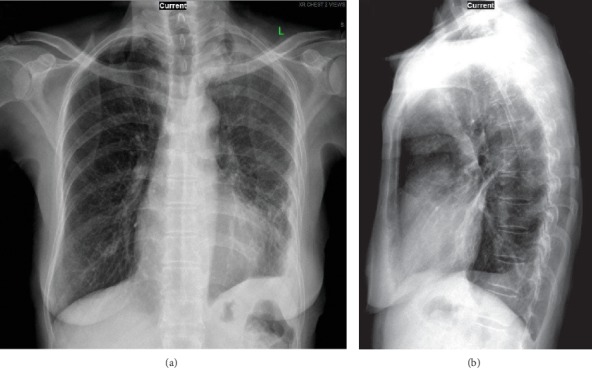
Chest X-ray images of the improvement of the opacity at the left lower hemithorax at week 3 of intravenous ertapenem therapy. Frontal view (a) and lateral view (b).
